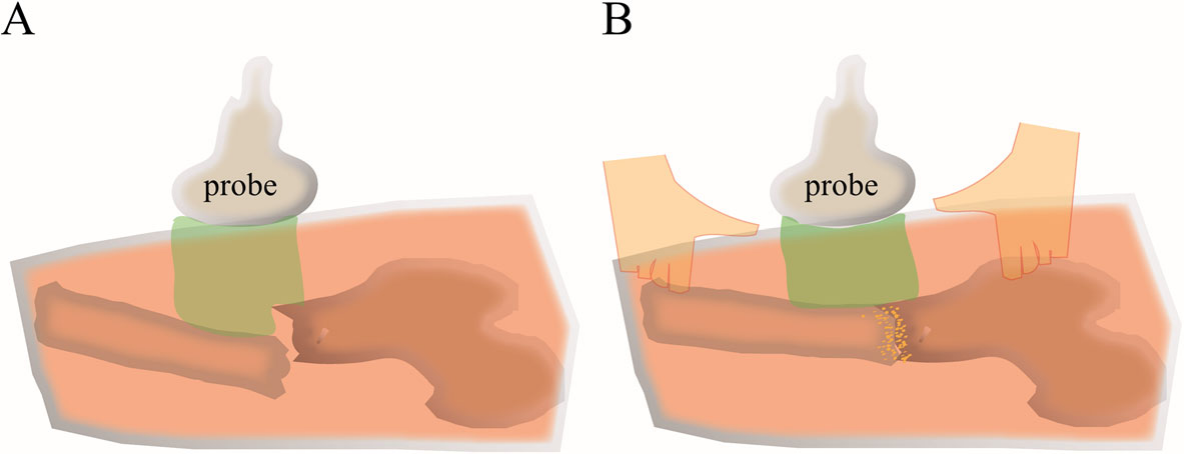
Closed reduction of FSF under surveillance US. a Checking the FSF using the ultrasound scanner. b Ultrasound surveillance and assistance for closed reduction of the FSF. FSF, femoral shaft fracture; US, ultrasonography
